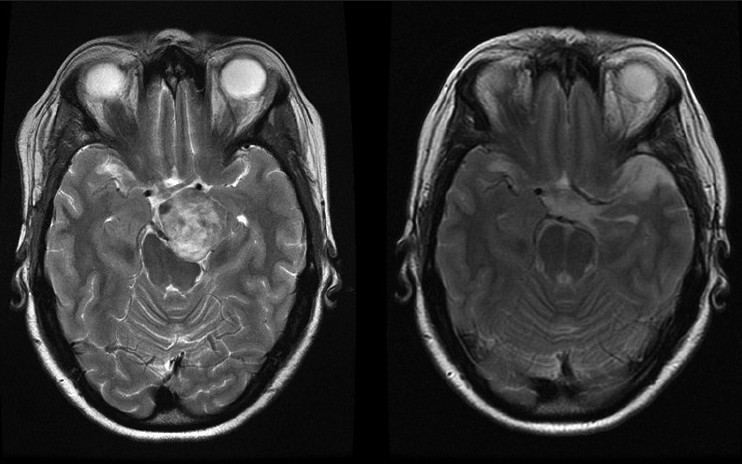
A preoperative (a) and a postoperative (b) T2-weighted axial MRI scan. The preoperative scan (a) shows a well-defined cerebrospinal fluid cleft around the mass
